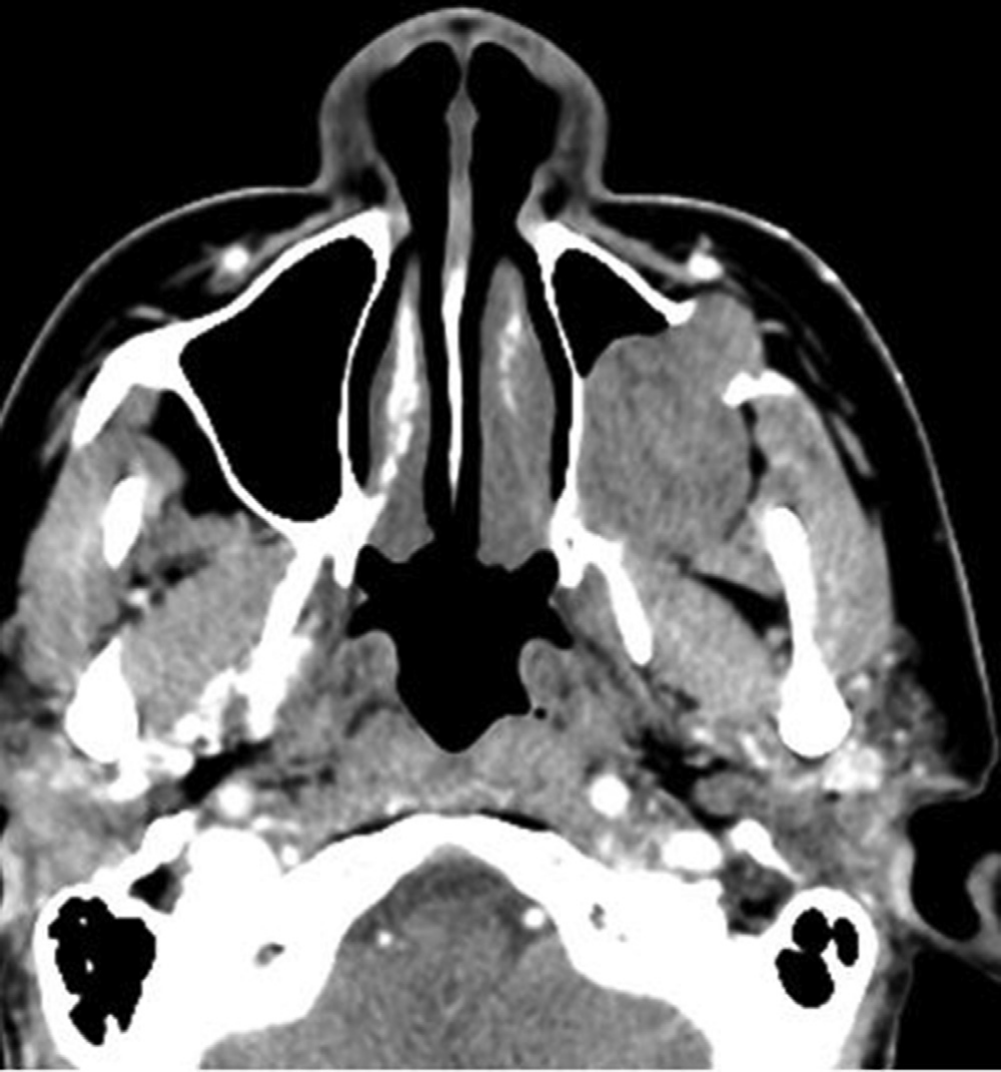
Axial CT post contrast: Soft tissue density mass involving the left maxillary sinus with associated bony erosion of the anterior and lateral walls.
